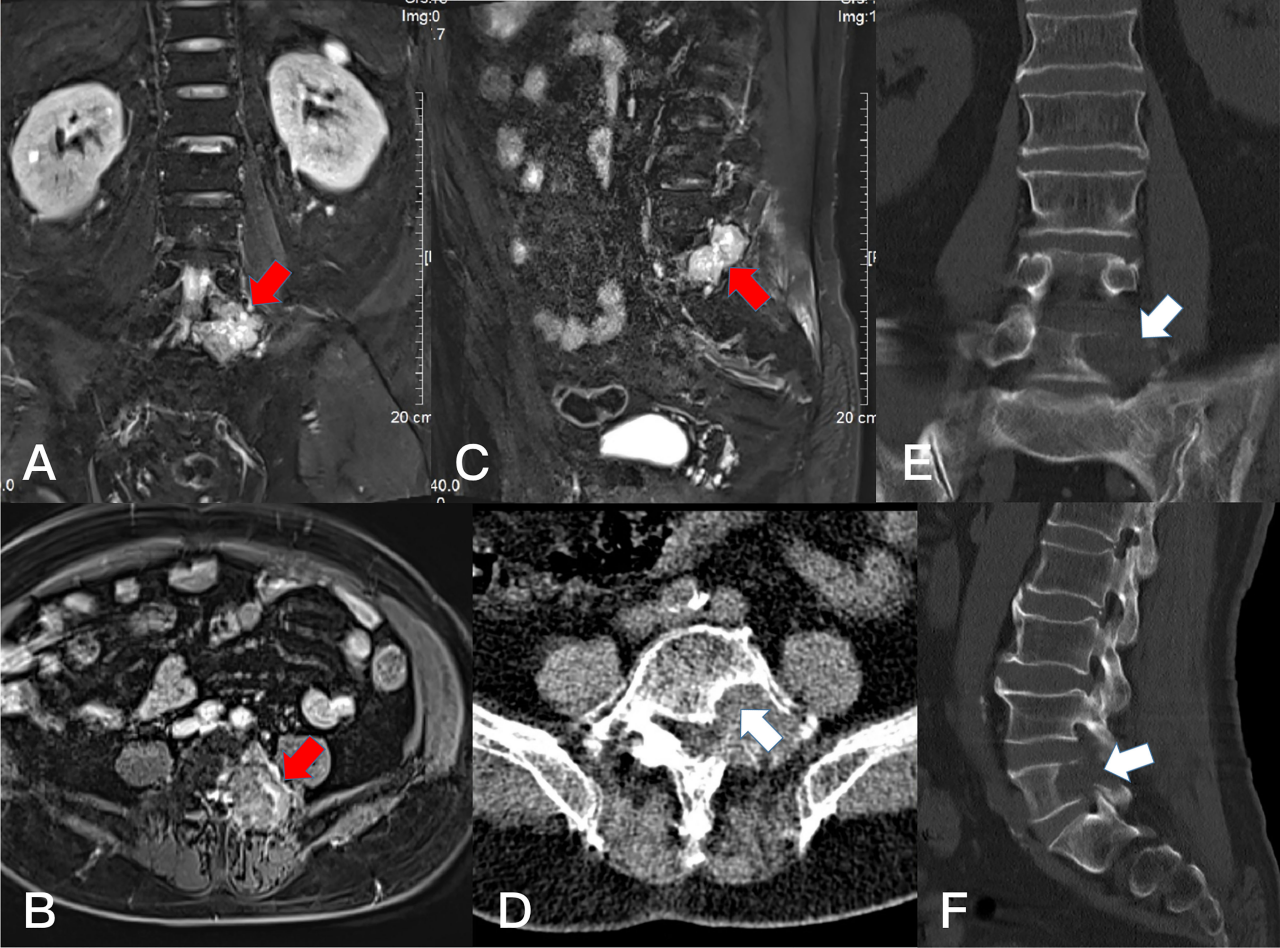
Preoperative T1-weighted enhanced MRI on coronal (A) plane, axial (B) plane, and sagittal (C) plane, revealing a dumbbell tumor (Eden III) in the left L5-S1 foramen (red arrows). The CT scan on axial (D) plane, coronal (E) plane, and sagittal (F) plane showed the bone damage of left lamina, facet joint and vertebral body of L5(white arrows).
